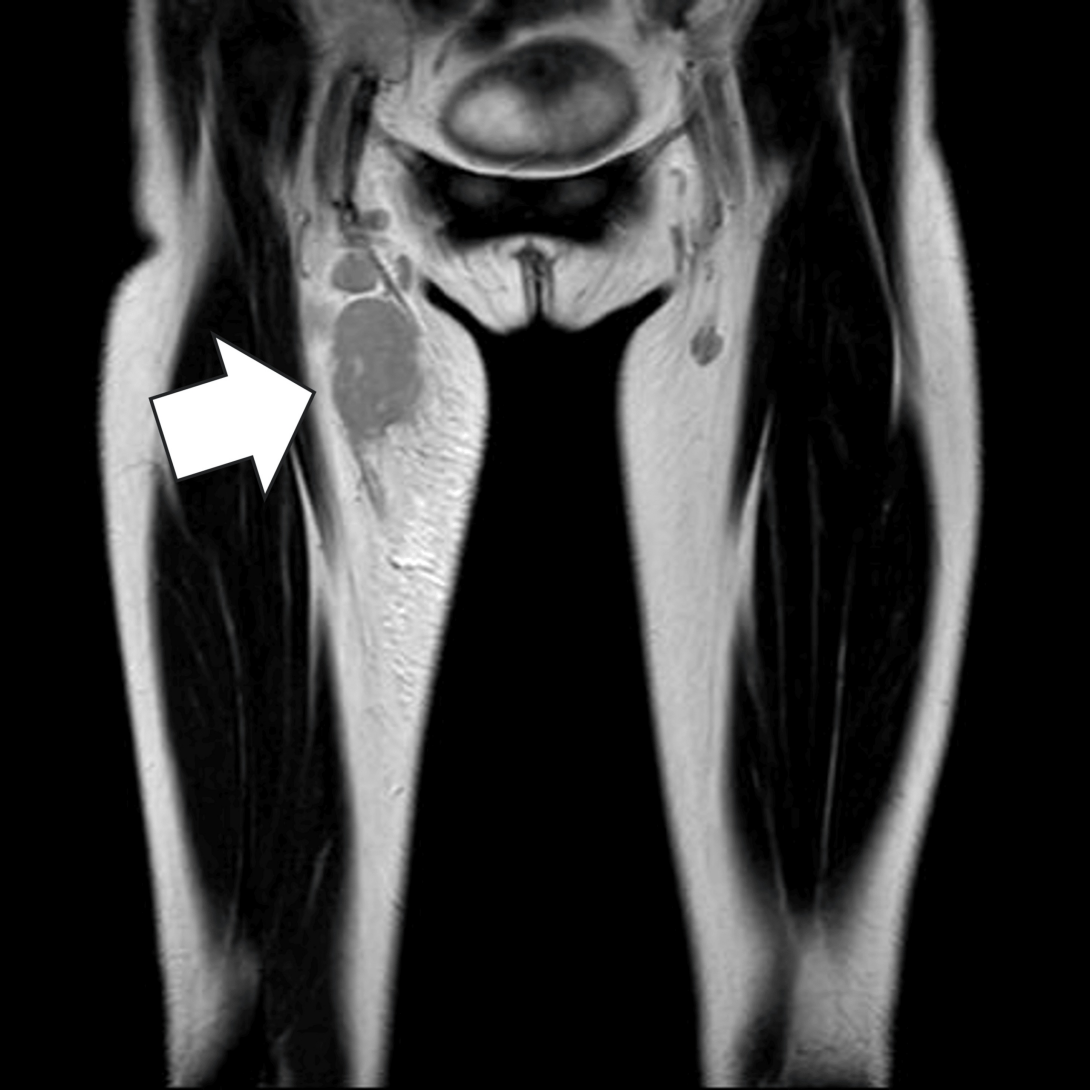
MRI findings of an enlarged lymph node in the anterior right thigh with inguinal lymphadenopathy. Coronal T2-weighted MRI of the pelvis and thighs demonstrating an enlarged, hyperintense lymph node in the subcutaneous tissue of the anterior right thigh, with additional enlargement of inguinal lymph nodes; the white arrow indicates the lesion. Surrounding soft tissue edema is also present.
